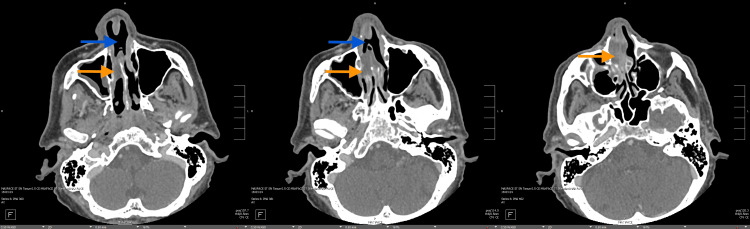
CT maxillofacial without contrast (axial) showed a large nasal septal perforation with mucosal thickening and hyperenhancement along the nasal septum (blue arrow) Evidence can also be seen of an associated lobulated fluid density centered along the nasal septum at the superior and posterior margin of septal perforation extending into the right nasal cavity toward the middle meatus, which was concerning for a nasal septal abscess (orange arrow)
